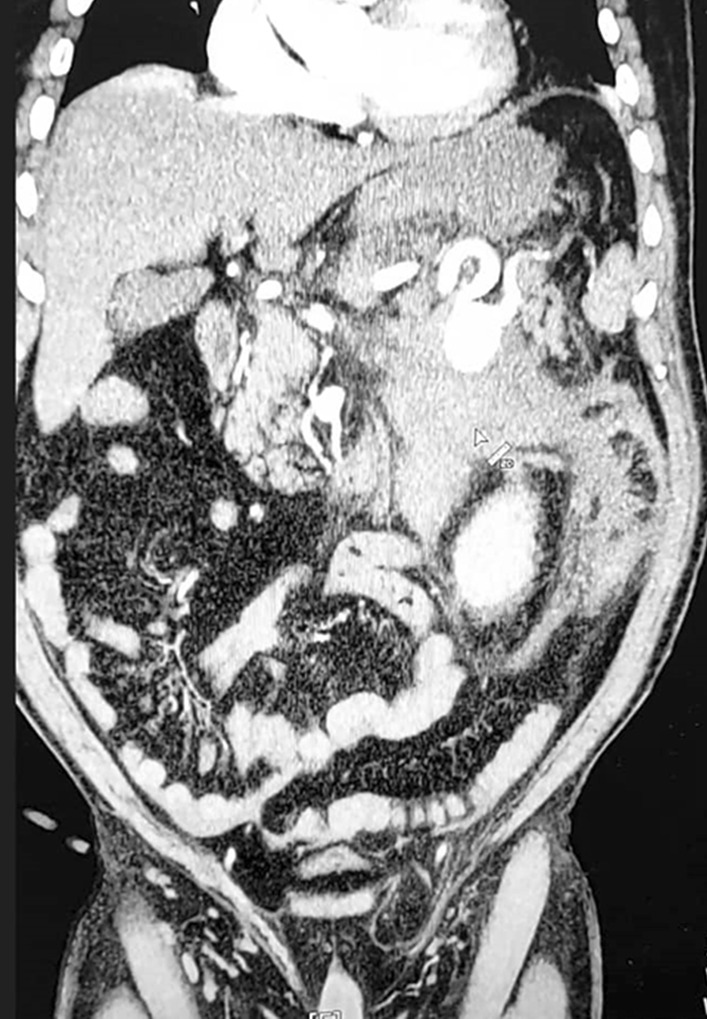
Computed tomography scan of a 59-year-old man with a ruptured splenic aneurysm
